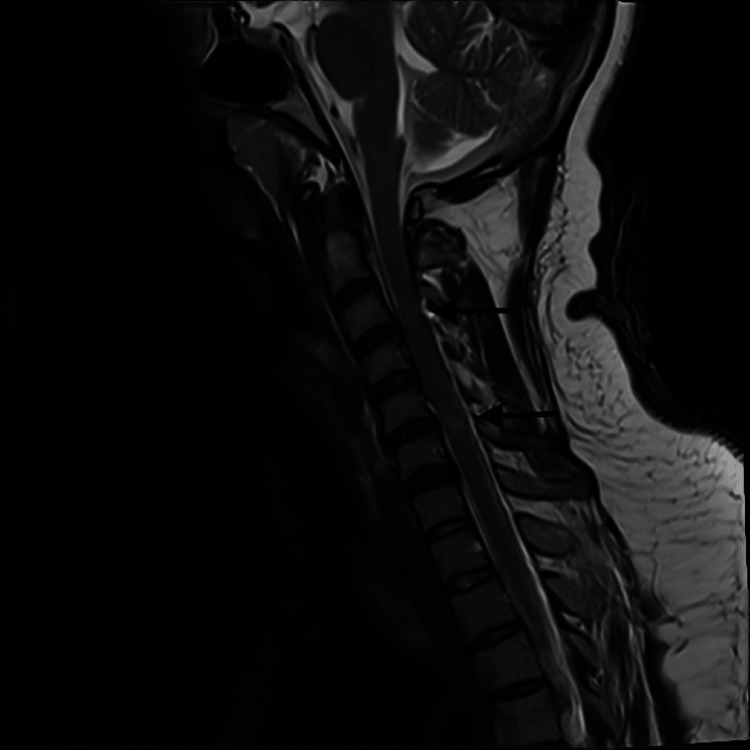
MRI image showing spinal cord injury at C3-C4 and C5-C6 levels
